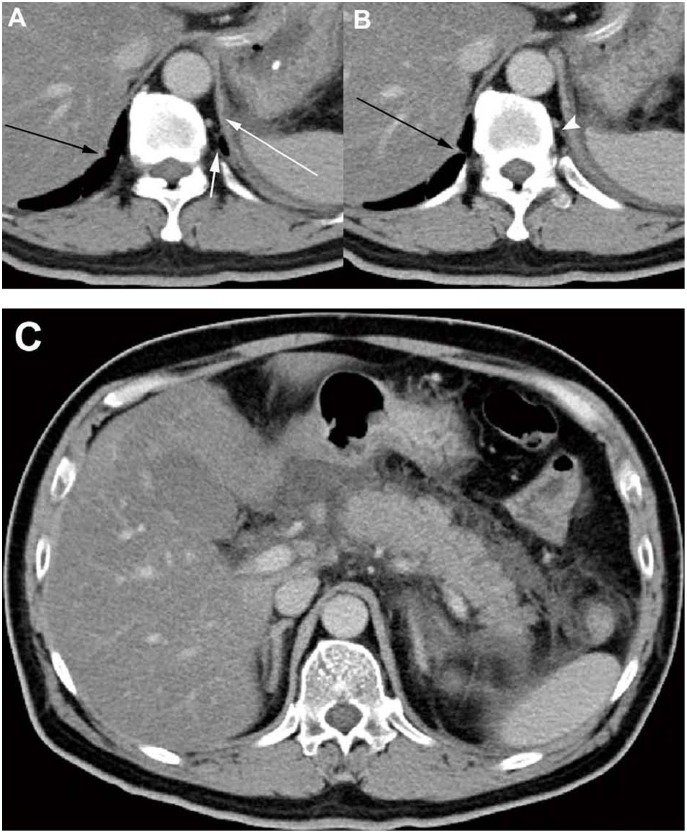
CT manifestations of the retrocrural space involvement in a 48-year-old man with AP. (A, B) CT scans obtained 3 days after admission showed acute fluid collections at the left subphrenic spaces. They resulted in the hydropsia of the left diaphragm (*long white arrow*), and the thickening of the left mediastinal pleura (*short white arrow*). In addition, a streaky density occurred at the left retrocrural space (*arrowhead*). On the opposite side, the right crus has a rough edge (*black arrow*). (C) CT scans displayed the peripancreatic fluid collections had extended into the left retroperitoneal space.
